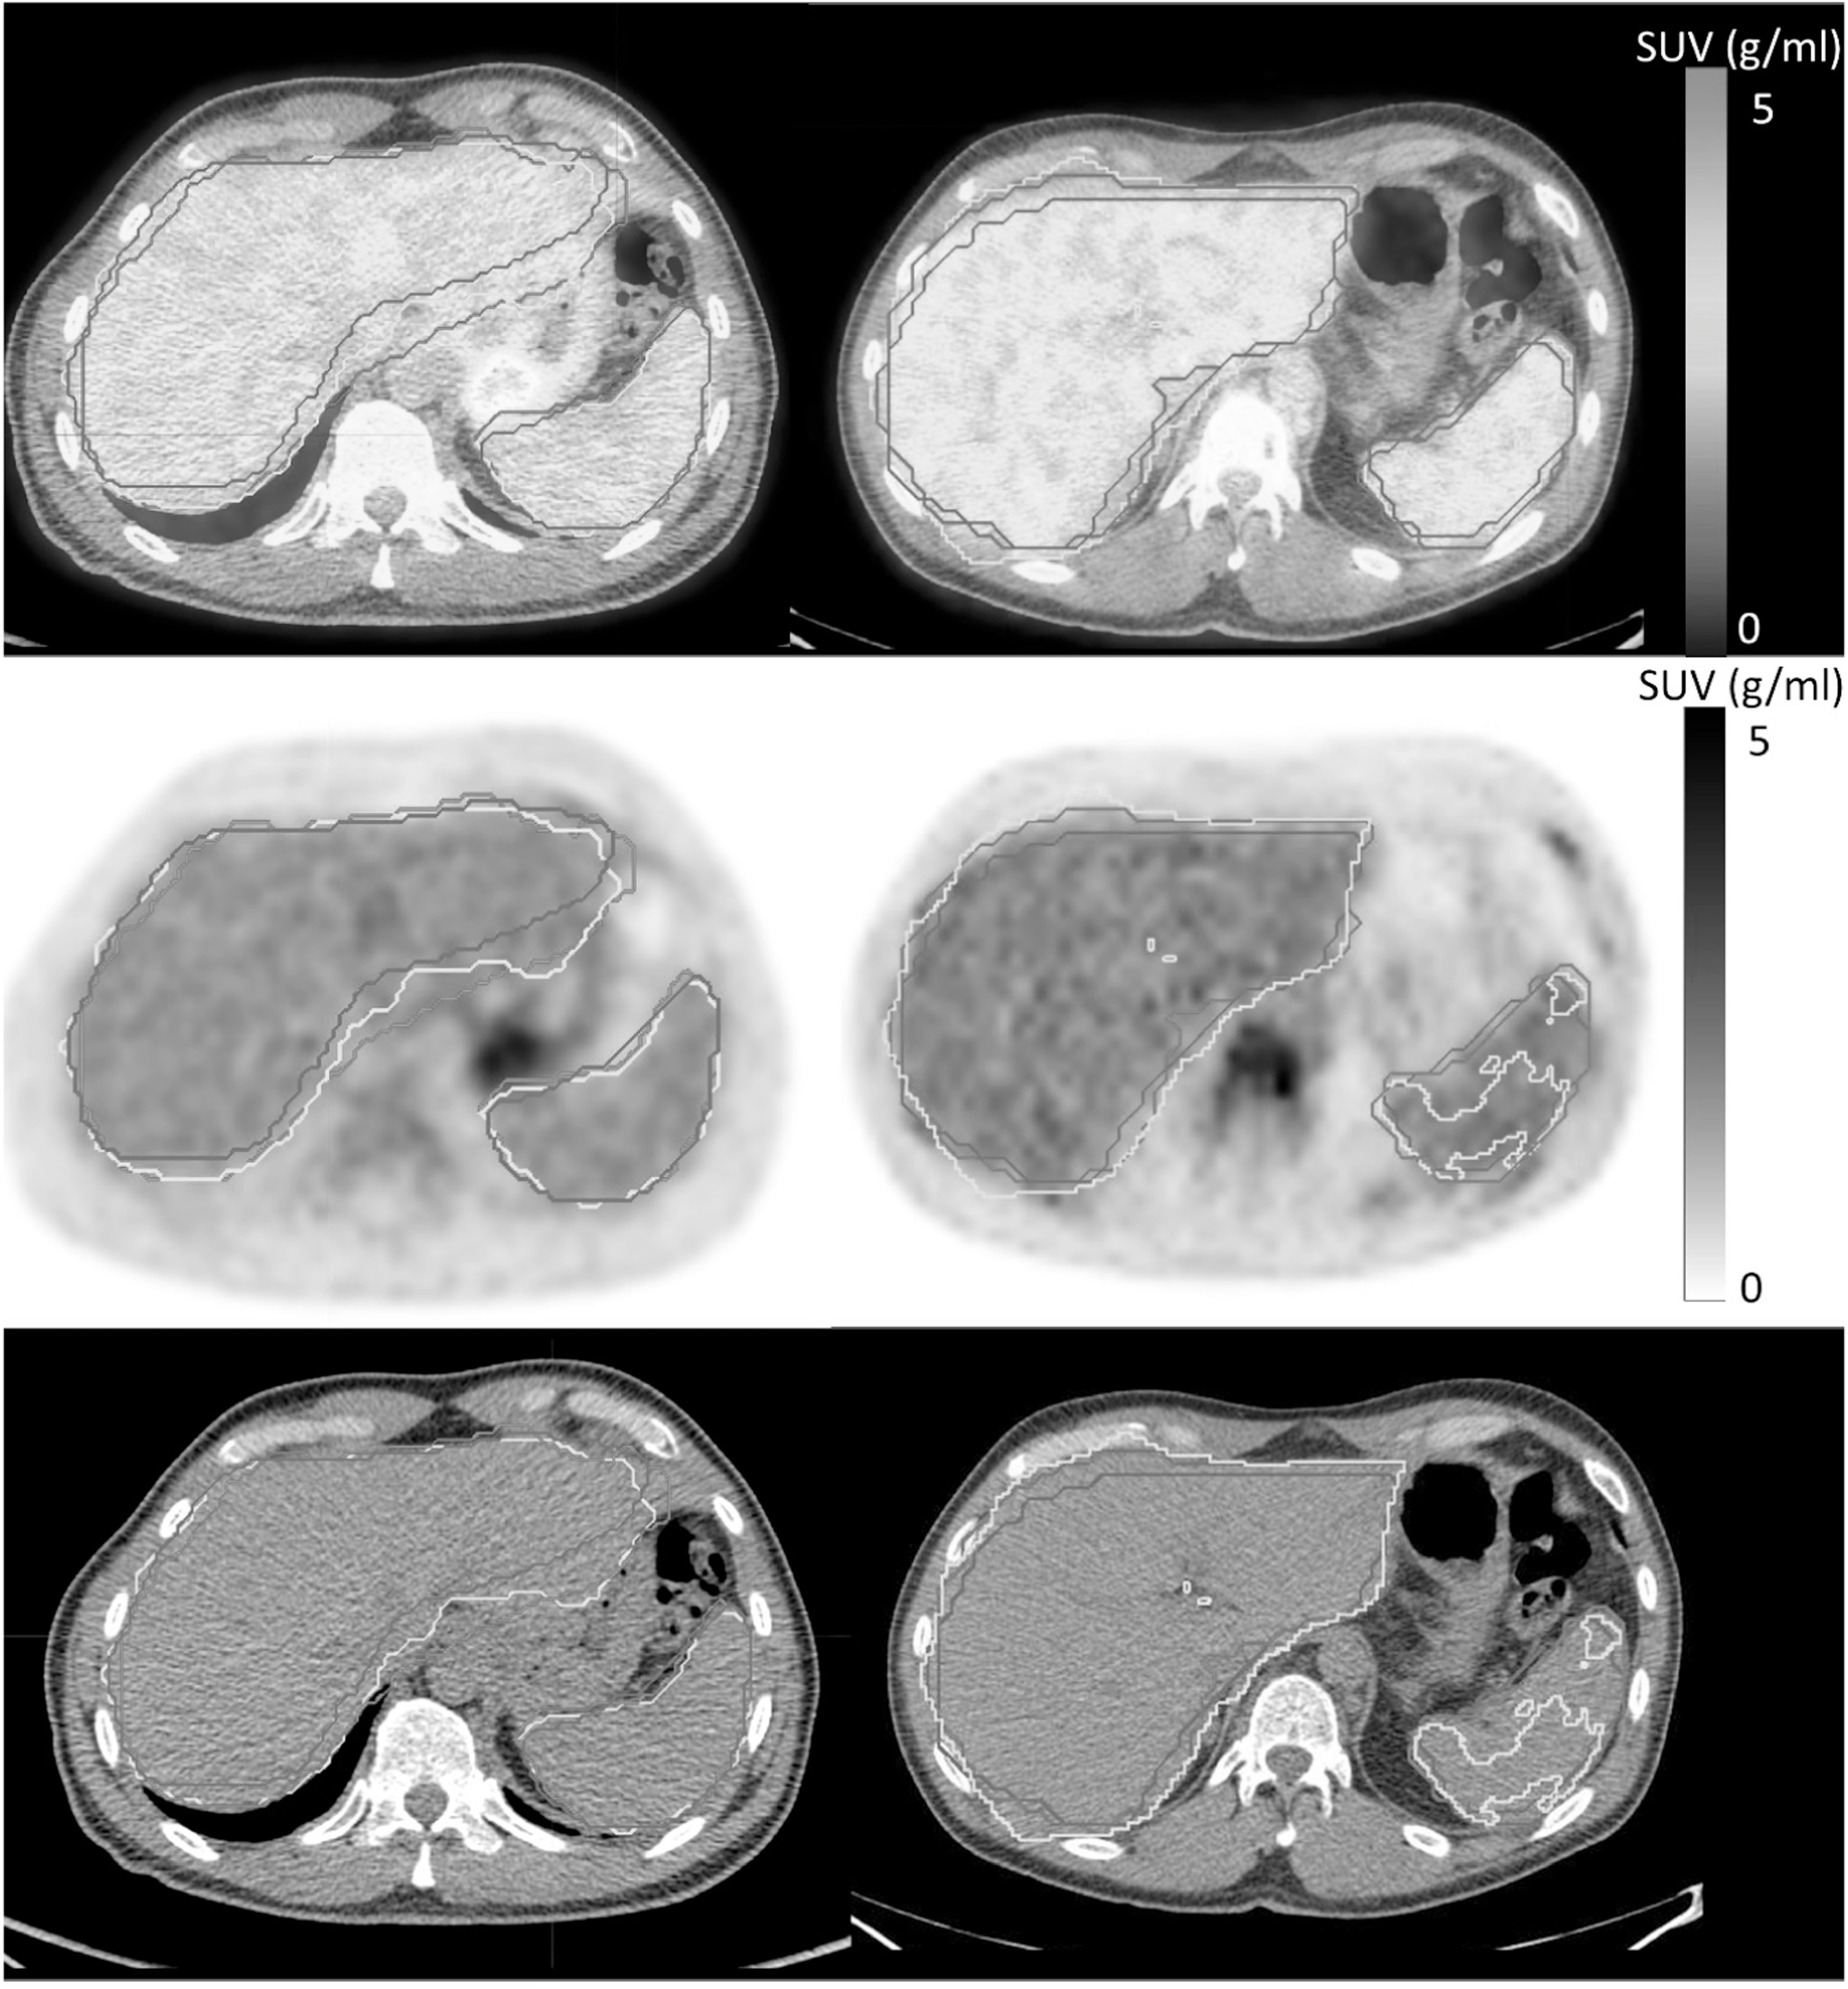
PET-CT fused images (first row) along with corresponding PET only (second row) and CT only images (third row) from two patients. The automated methods provided similar contours for the liver and spleen in patient 1 (left column) but failed to accurately segment organs in patient 2 (right column). The brown contour represents PET-man, the yellow CT-DL and the magenta the CT-Atlas.
